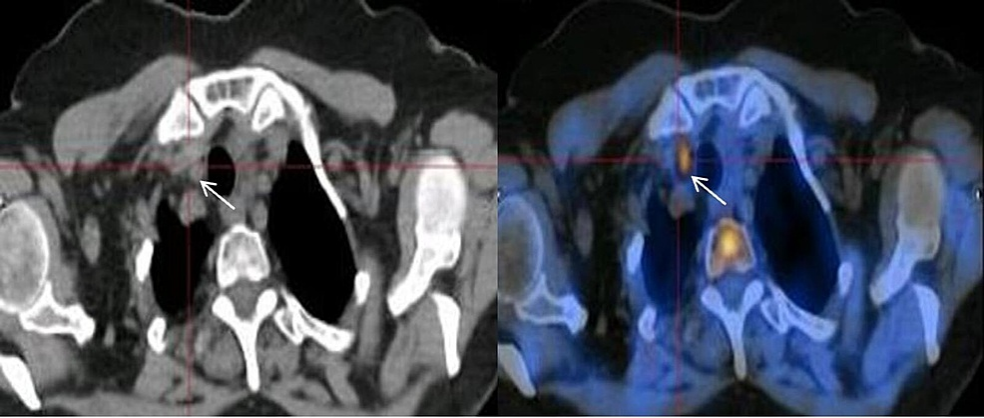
Fluorine-18-Fluorocholine positron emission tomography/computed tomography White arrows – prominent avidity in the posteroinferior region of the right thyroid lobe.
